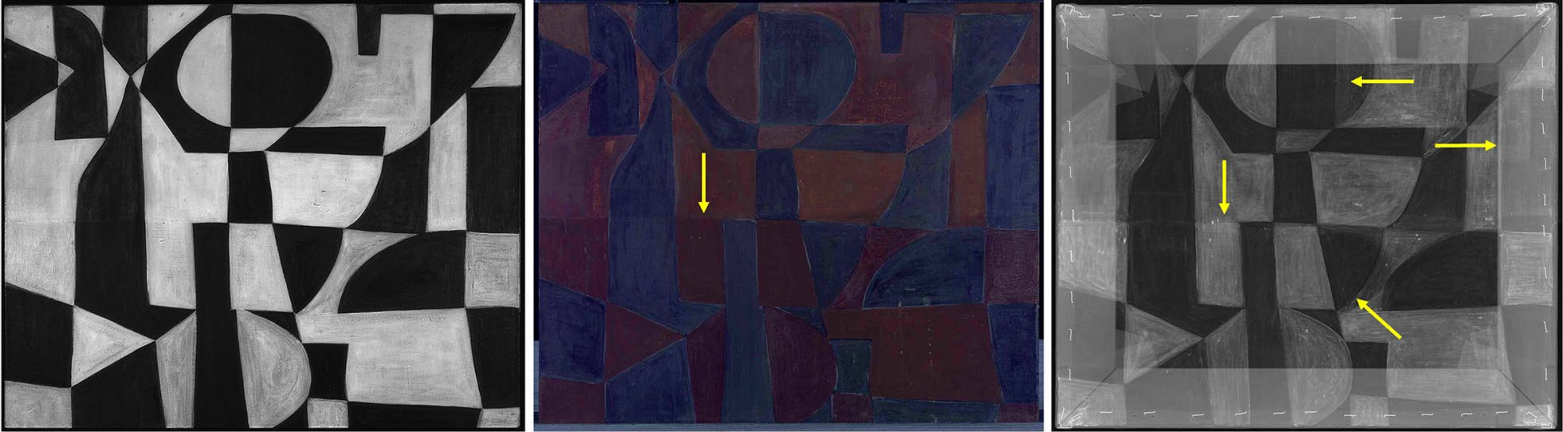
From left to right: IR reflectogram, UV photograph, and X-radiograph of *Iberia #25* (1948). Arrows indicate compositional changes and varying paint opacities discussed in the text
